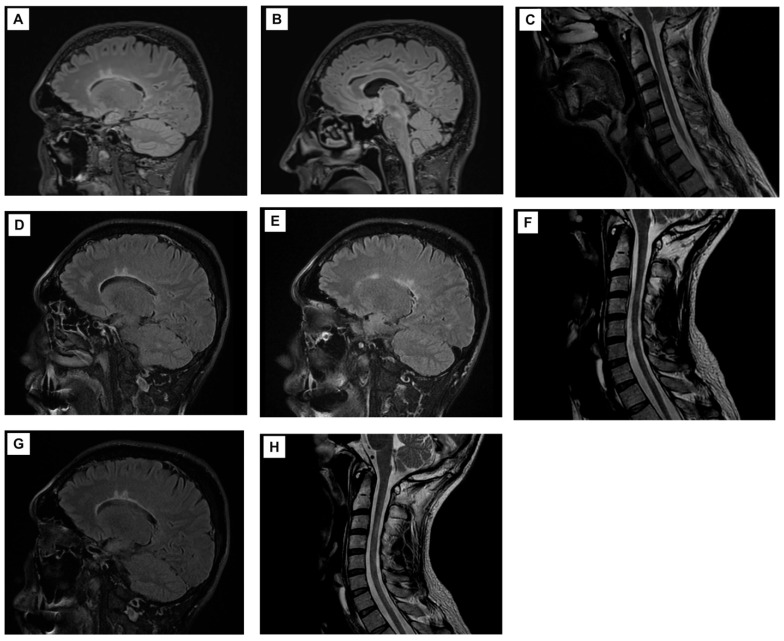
Serial Sagittal MRI. (A) 2022—Brain FLAIR: Hyperintense demyelinating lesions perpendicular to the lateral ventricles. (B) 2023—Brain FLAIR: Persistent periventricular demyelinating lesions and new cerebellar lesion. (C) 2023—Cervical Spine T2: No demyelinating lesions identified. (D,E) 2024—Brain FLAIR: Stable periventricular and cerebellar lesions with new subcortical demyelinating lesion. (F) 2024—Cervical Spine T2 frFSE: Hyperintense lesions at C3–C4 and C5–C6 levels. (G) 2025—Brain FLAIR: Stable appearance of periventricular, cerebellar, and subcortical lesions. (H) 2025—Cervical Spine T2 frFSE: Stable cervical cord lesions with no new lesions detected.
